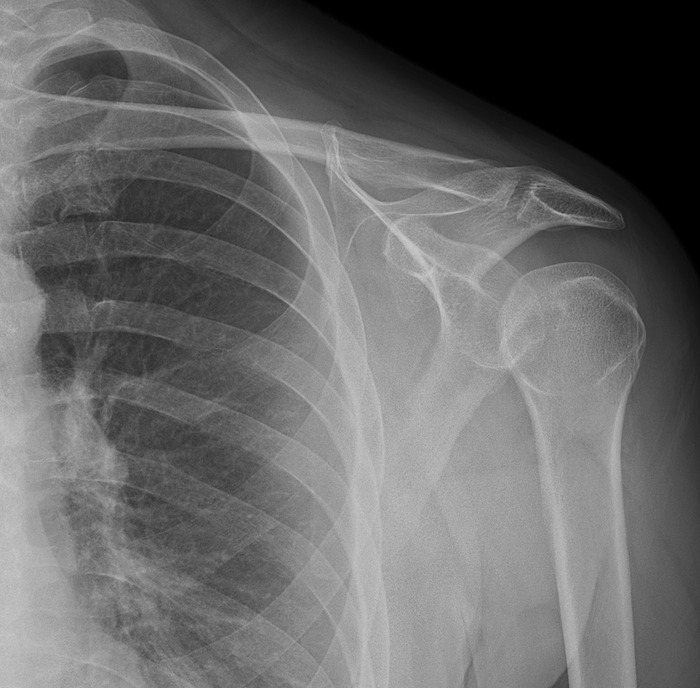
Conventional radiograph of the left shoulder demonstrated no acute pathology.
